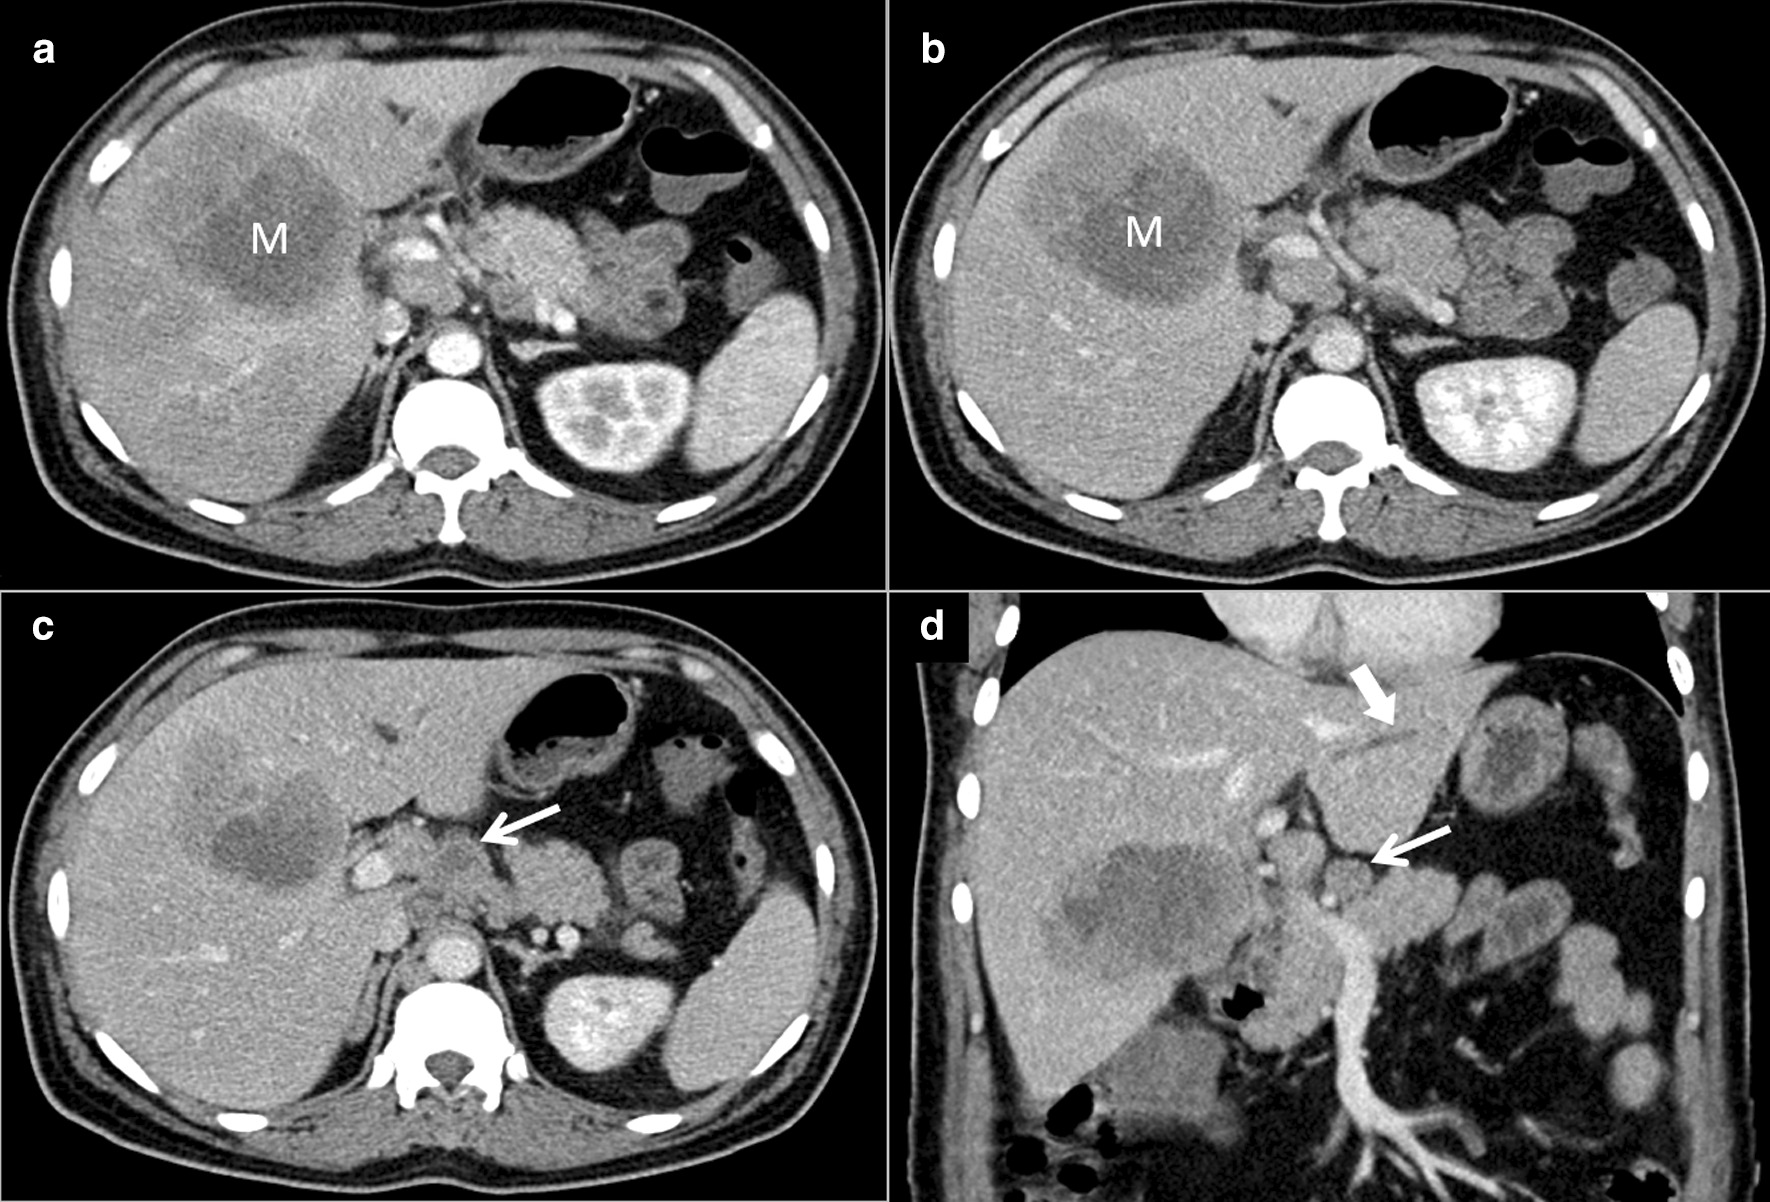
Contrast-enhanced abdominal computed tomography images acquired on initial presentation (a axial image in the arterial phase; b, c axial images in the venous phase; and d coronal image in the venous phase) demonstrating a heterogeneous mass (M) with internal hypodensity in segment V (a and b). Necrotic lymph nodes (thin arrows in c and d) and mild biliary dilatation (thick arrow in d) were noted
